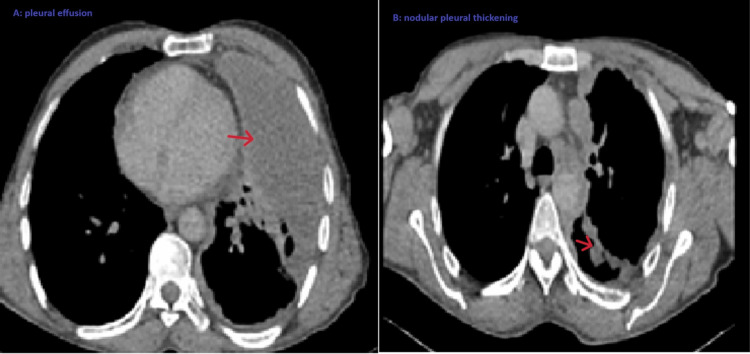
A chest CT scan with mediastinal window revealed nodular pleural thickening on the left side, associated with a moderate left pleural effusion and mediastinal lymphadenopathy. A: The red arrow shows the pleural effusion. B: The red arrow shows the nodular pleural thickening.
